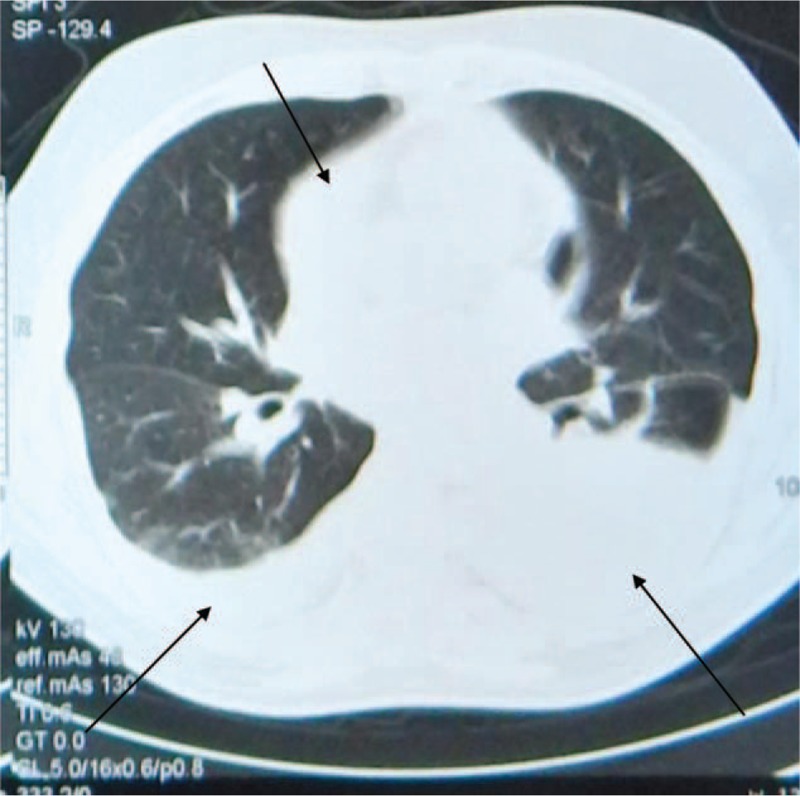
Chest-computed tomography. Pericardial and pleural effusions marked by arrows.
